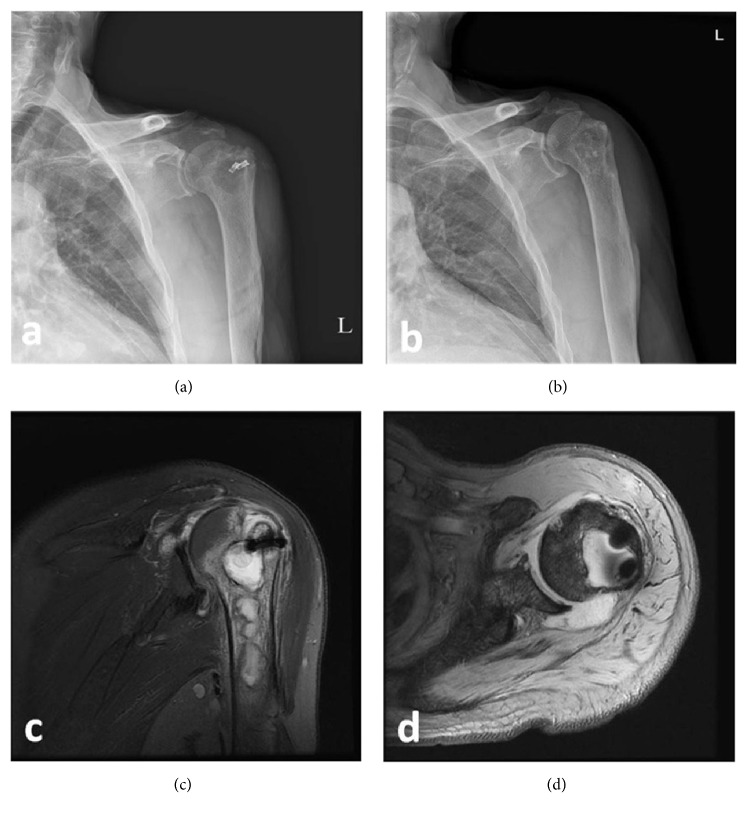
Radiographic evaluation of a 70-year-old man with a positive culture. (a) Preoperative standard X-ray (anterior-posterior) view revealing metallic anchors with an osteolytic lesion on the greater tuberosity, (b) postoperative healed bone lesion, (c) MRI-T2-coronal view showing characteristics of osteomyelitis with a multilobulated bone marrow lesion, edematous change, and synovial thickening, and (d) MRI-T2-axial view showing fluid collection in the humeral head, diffuse synovial thickening, and two metallic anchors at the greater tuberosity.
